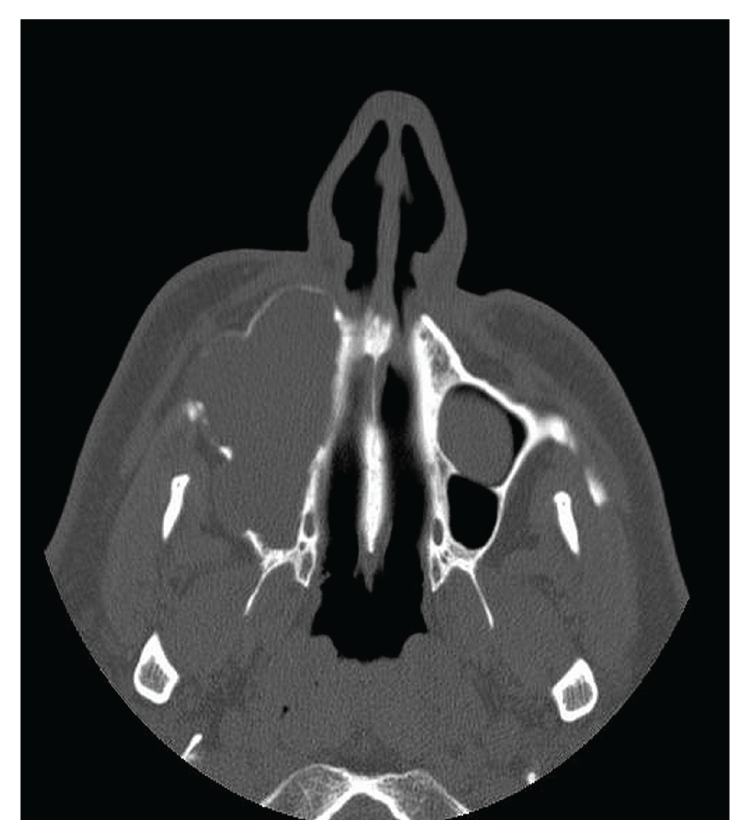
Axial Computed Tomography (CT) reveals expansion of the walls of the right maxillary sinus, obstruction with low density tissue of the whole cavity, and local erosion of the walls.
